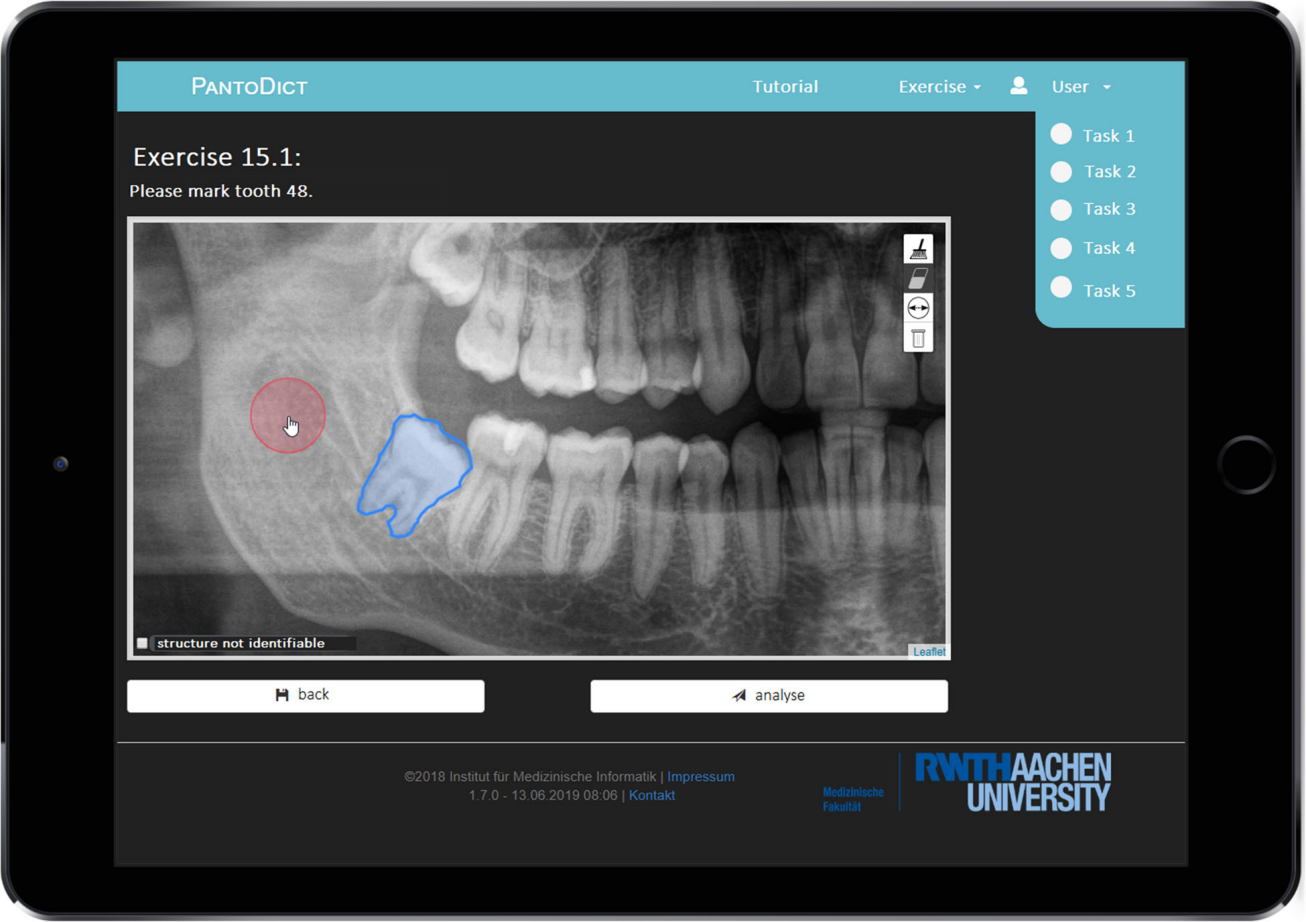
Screen view showing a marking exercise in process. There are always 5 tasks for each radiograph. In this example, the participant is asked to mark the tooth 48
